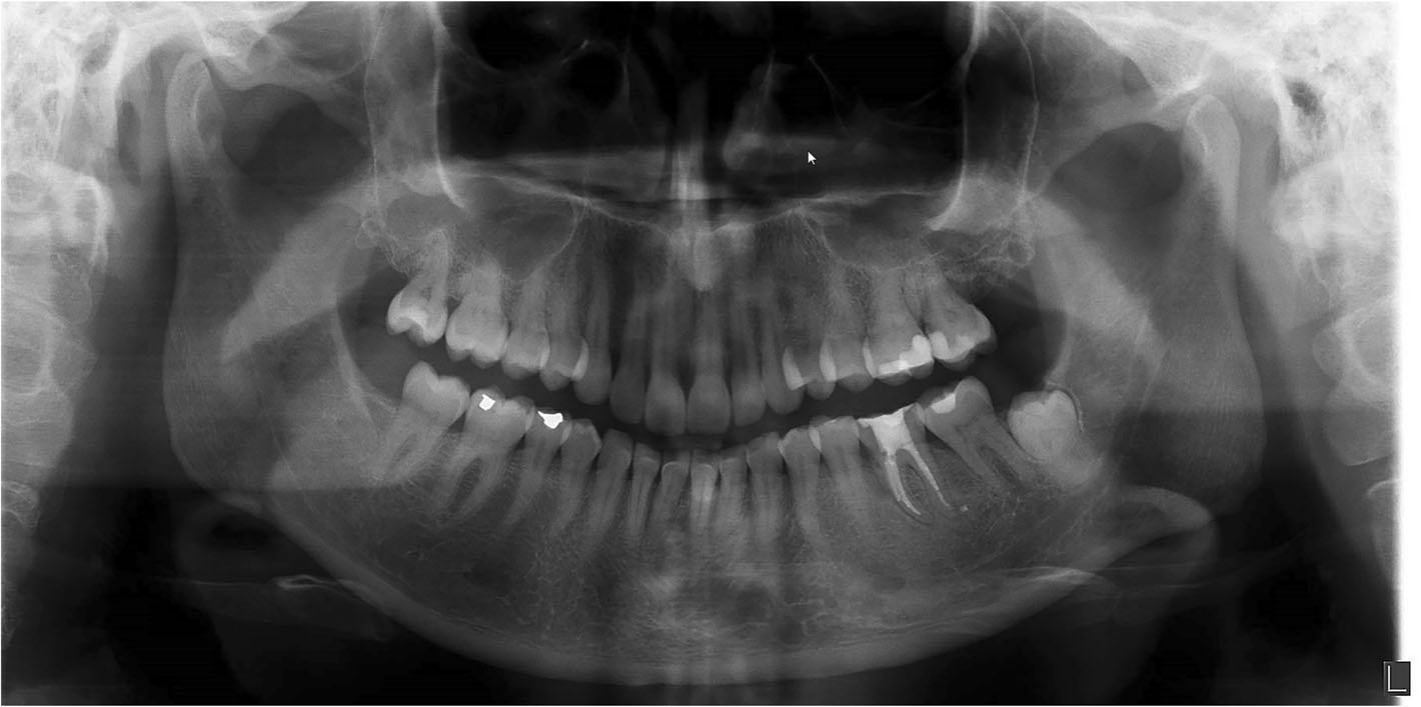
Pre-operative panoramic radiograph of a failing molar in position 36
